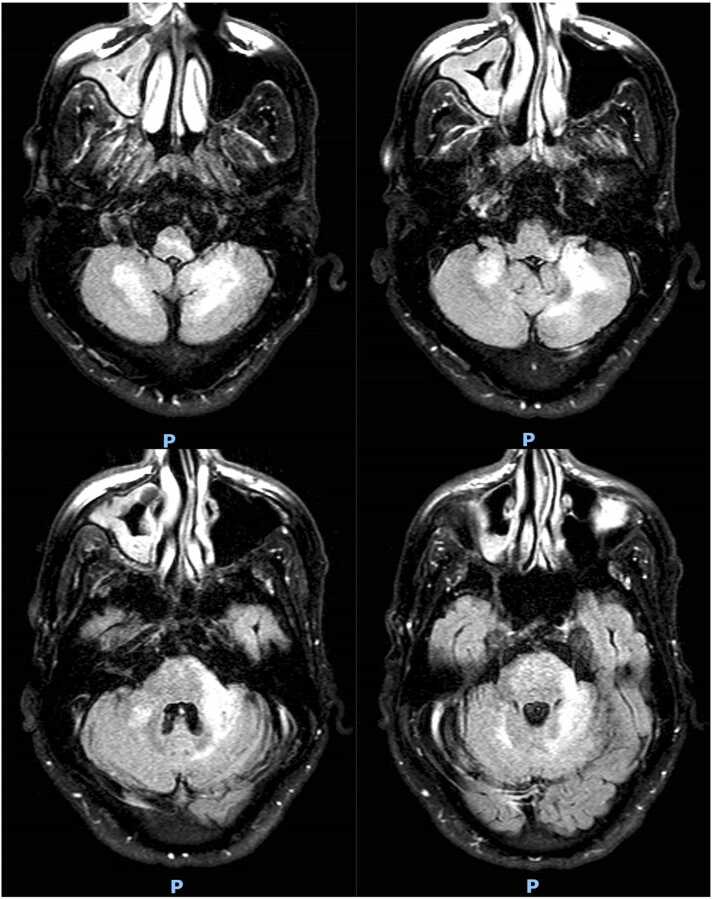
MRI Brain with and without contrast; axial T2 FLAIR (April 7, 2022). Stable geographical signal abnormalities of the posterior fossa compatible with the clinical diagnosis of PML. Stable additional nonspecific multifocal, overall mild signal changes of the supratentorial white matter.
